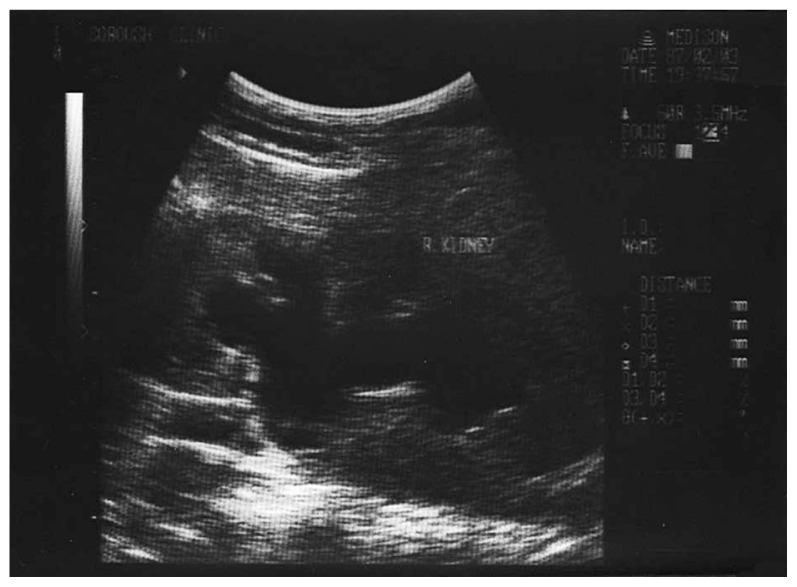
Bladder sonography is consistent with a neuorogenic bladder.
